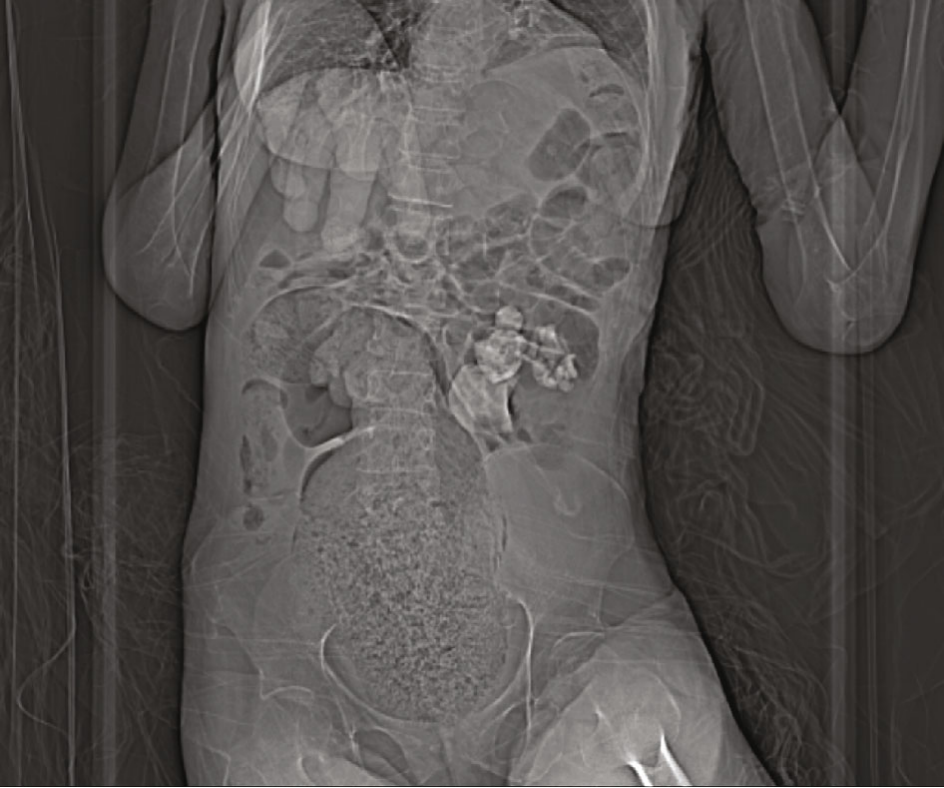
Initial CT scan scout film, showing the staghorn calculus located in the left kidney.
